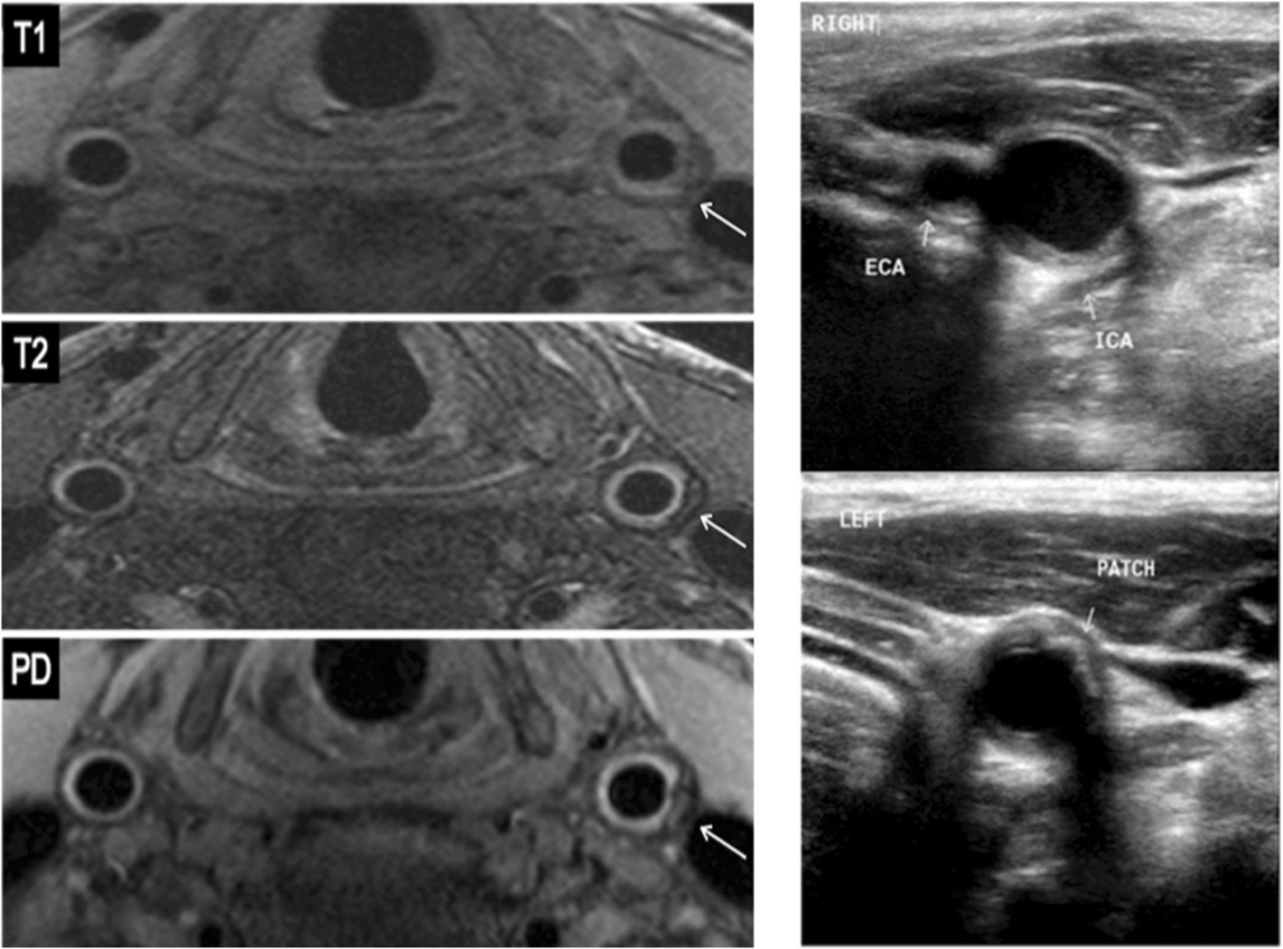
Patient with bilateral carotid endarterectomy five and a half years previously (2038 days); T1, T2 and PD weighting of both common carotid arteries shown (left). The right common carotid artery demonstrates a normal post-operative appearance, both on CMR and ultrasound (top right). However, a 30% restenosis of the left common carotid was noted on ultrasound (bottom right), which was seen as a crescent of predominantly low signal surrounding the artery on CMR (white arrows).
